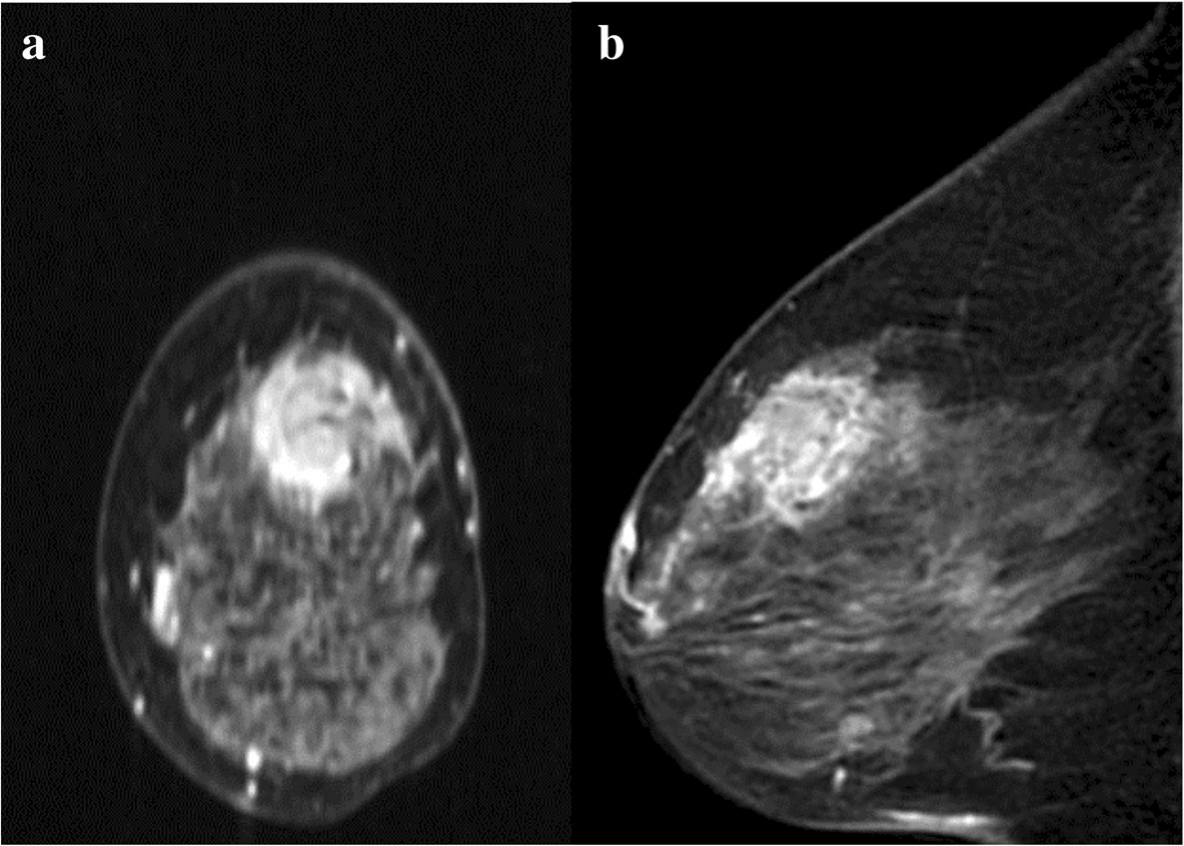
Magnetic resonance imaging (MRI) showed enhancement of a 17 × 17-mm indistinct mass surrounded with a non-mass enhanced segmental lesion toward the nipple side spreading a maximum of 74-mm range. Coronal view (a). Sagittal view (b)
